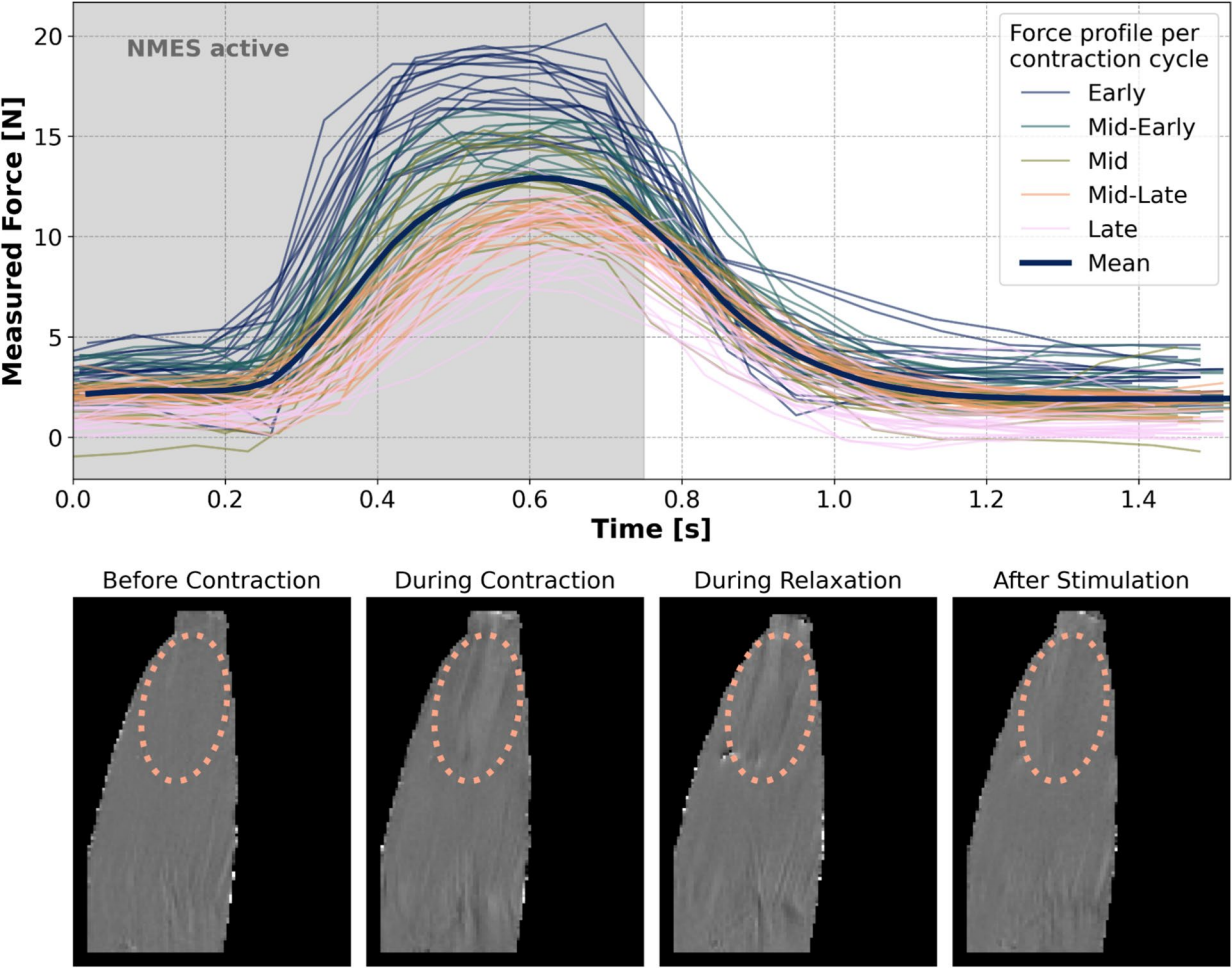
Measured force evoked by NMES stimulation during a phase-contrast acquisition per NMES cycle, upper panel. Each NMES cycle consists of 0.78 s on-time with 300 us pulses at 60 Hz (indicated by grey shading), followed by 0.78 s off-time. To make up for the averaging effects due to the temporal resolution of our device and trigger recordings, we subtracted a 0.05 s delay from our signal to improve the alignment of the force profiles per contraction. In total, 93 contractions were recorded, shown above with five different colours to distinguish early contractions at the beginning of the phase-contrast MRI acquisition from late contractions towards the end of the acquisition. The bottom panel shows velocity images from the phase-contrast MRI sequence showing the dynamic changes in the muscle: before contraction, during contraction, subsequent relaxation and final return to baseline after stimulation
